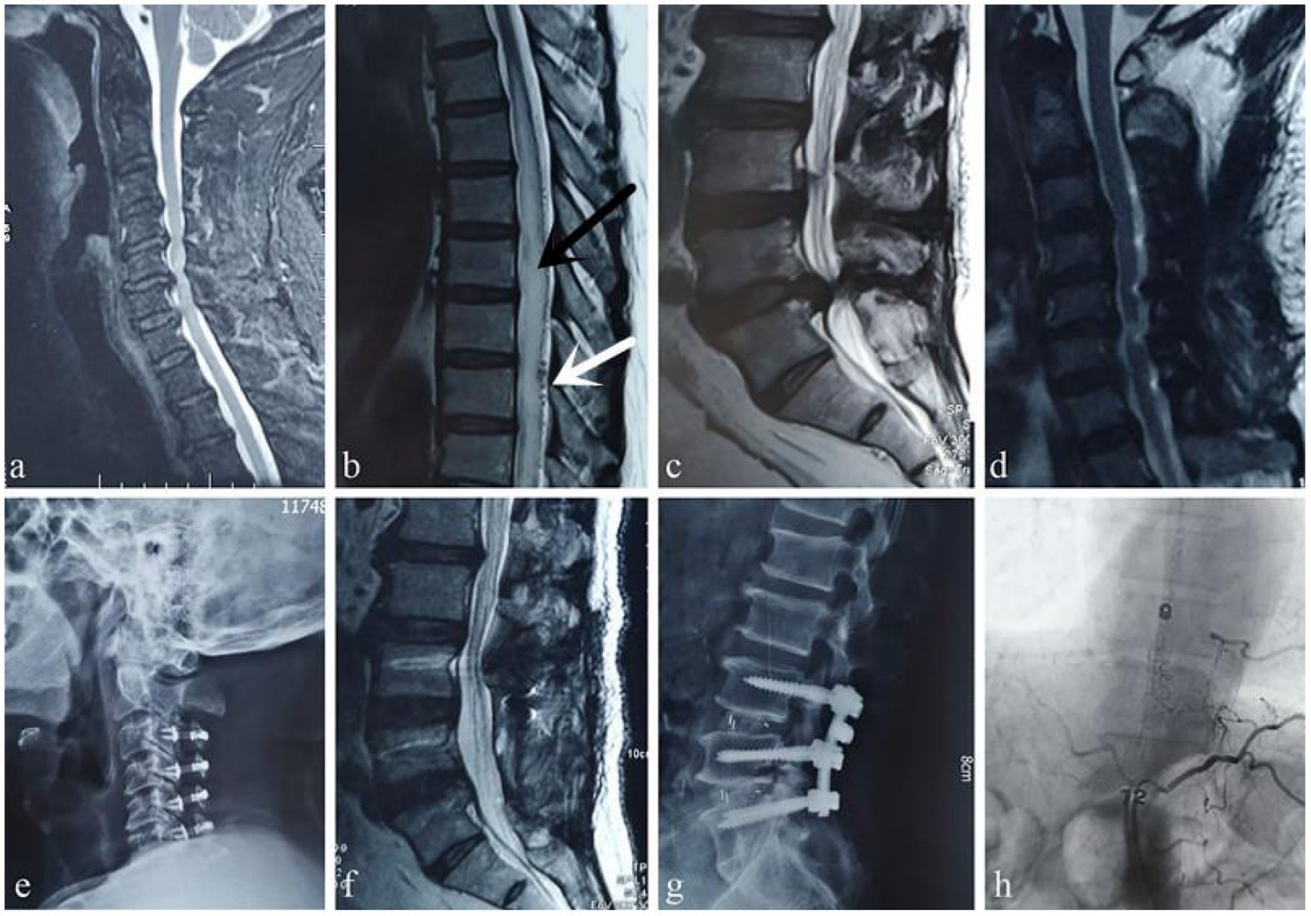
Case 3. A 59-year-old man was first evaluated at the orthopedic clinic because of “progressive lower extremity weakness and difficulty urinating for 2 month.” Cervical spine MRI shows spinal stenosis (a), edema and signs of flow voids in the thoracic spinal cord, which are typical manifestations of a DVF (b) (white arrow: “beaded” dilated flow void signals, black arrow: spinal cord edema), and lumbar spinal canal stenosis (c). However, the orthopedic surgeon ignored the diagnosis of a DVF; he first performed cervical spinal canal expansion and decompression (d,e) and then misdiagnosed the disease as cauda equina syndrome caused by lumbar spinal stenosis and performed lumbar spinal canal decompression (f,g). These interventions aggravated the disease. The patient presented at our hospital approximately 9 months after the surgeries. After MDT consultation, angiography performed by a neurosurgeon confirmed that the fistula was at the T9 level (h).
